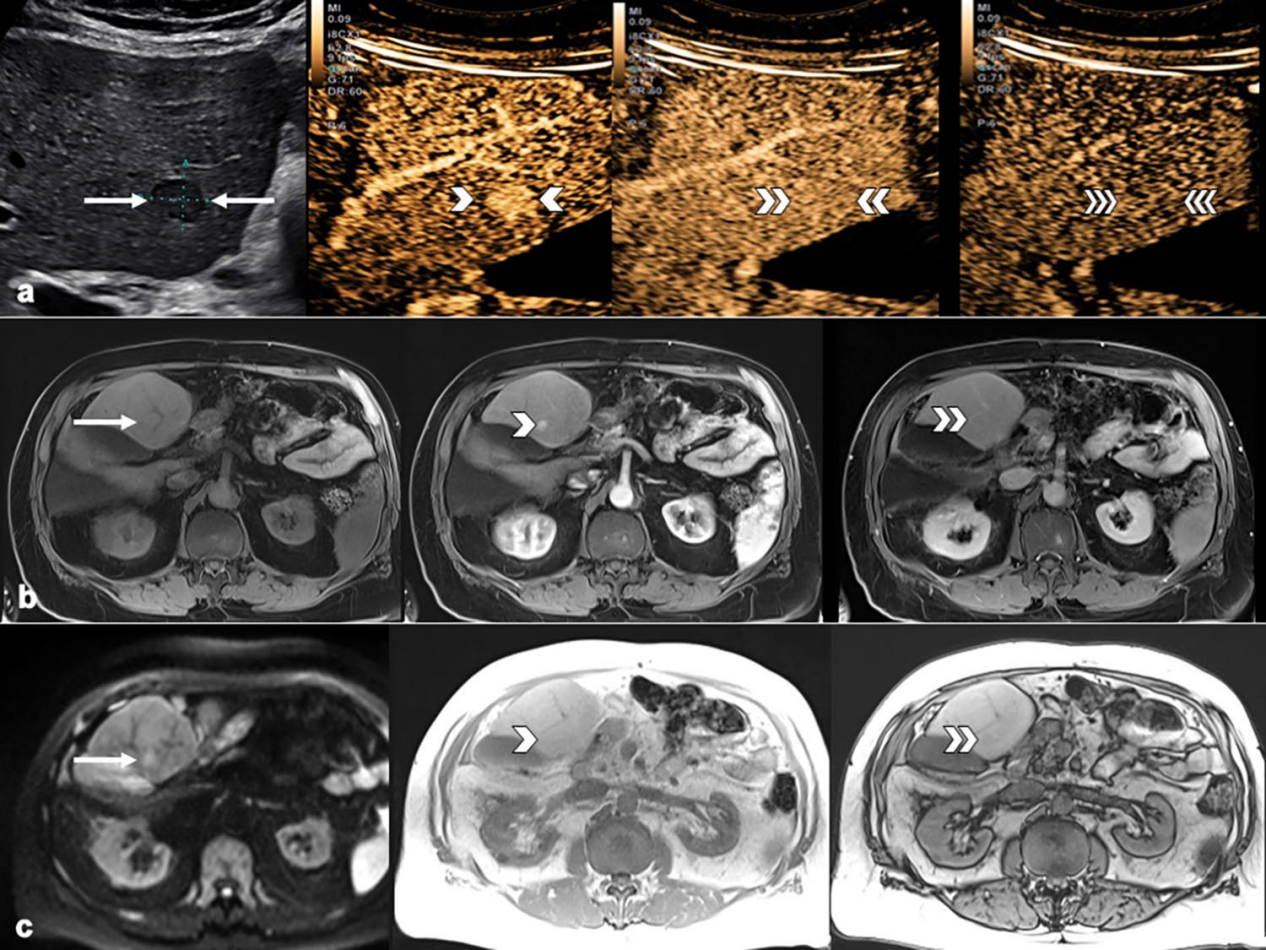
Combination of APHE present on CEUS and MRI in biopsy-confirmed HCC. a B-mode ultrasound demonstrating hypoechoic 1.4 cm mass in segment 4B of the left hepatic lobe (arrows). Arterial-phase image demonstrates hyper-enhancement (single arrowhead) with no contrast WO at 1 min (double arrowheads) or 4 min (triple arrowheads), resulting in CEUS LR-4 classification. The contrast agent used was Lumason/SonoVue. b Pre-contrast MRI image demonstrated ill-defined observation (arrow). Arterial-phase image demonstrated hyper-enhancement (single arrowhead) without WO on late-phase image (double arrowheads). c Observation demonstrates restricted diffusion (arrow). In-phase (single arrowhead) and opposed-phase (double arrowheads) images demonstrate no intralesional fat. This combination of image findings resulted in MRI LR-3 classification. APHE, arterial-phase hyper-enhancement; CEUS, contrast-enhanced ultrasound; MRI, magnetic resonance imaging; HCC, hepatocellular carcinoma; WO, washout
